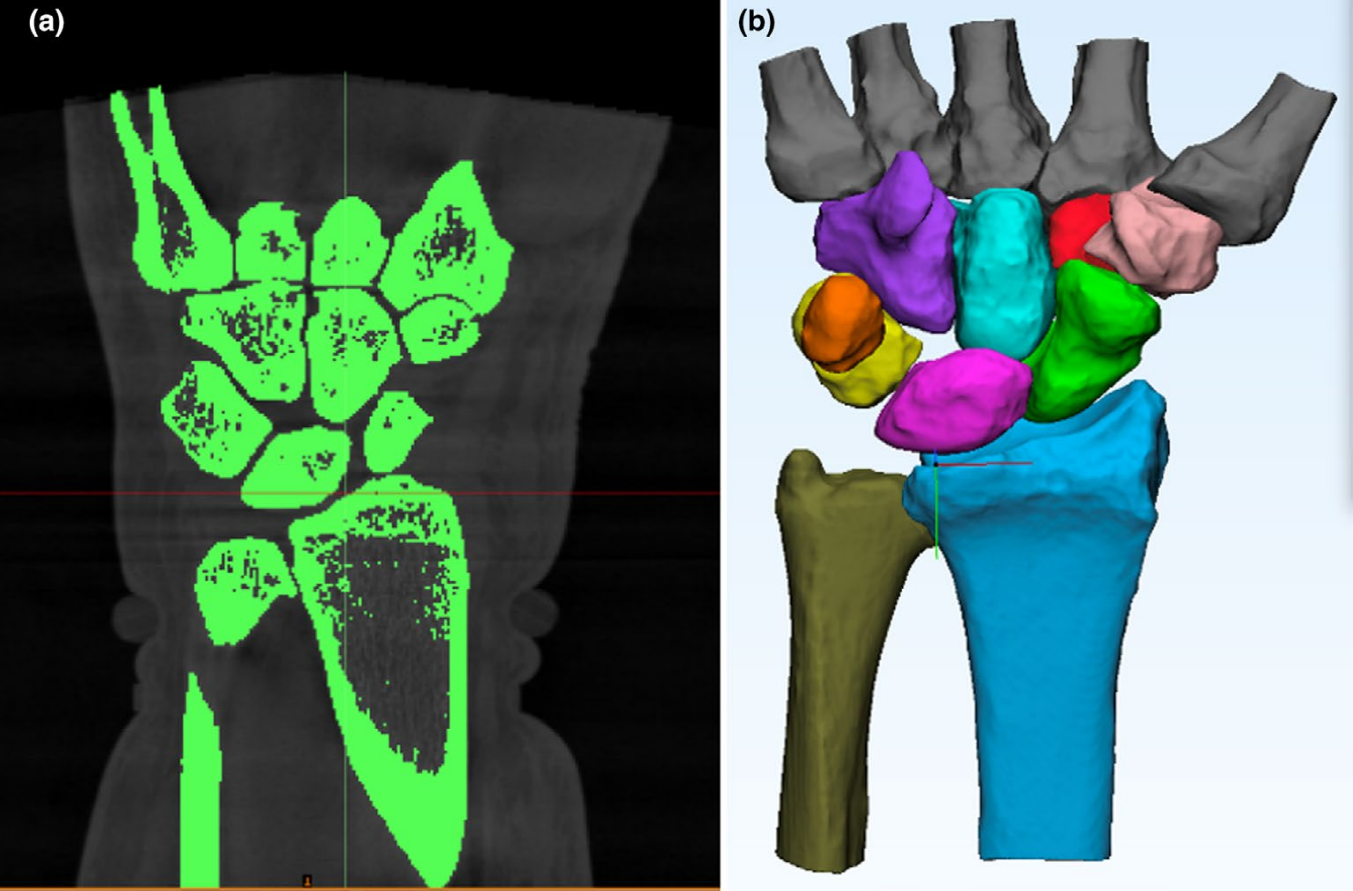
(a) The CT scans were processed to generate a 3D model by segmenting each individual bone. This involved the selection of individual bones, ensuring unambiguous identification within the program. This process was repeated for each bone to obtain a comprehensive 3D model of the wrist. (b) Subsequently, post‐segmentation tools such as smoothing and wrapping were employed to enhance the surface details. CT, computer tomography.
